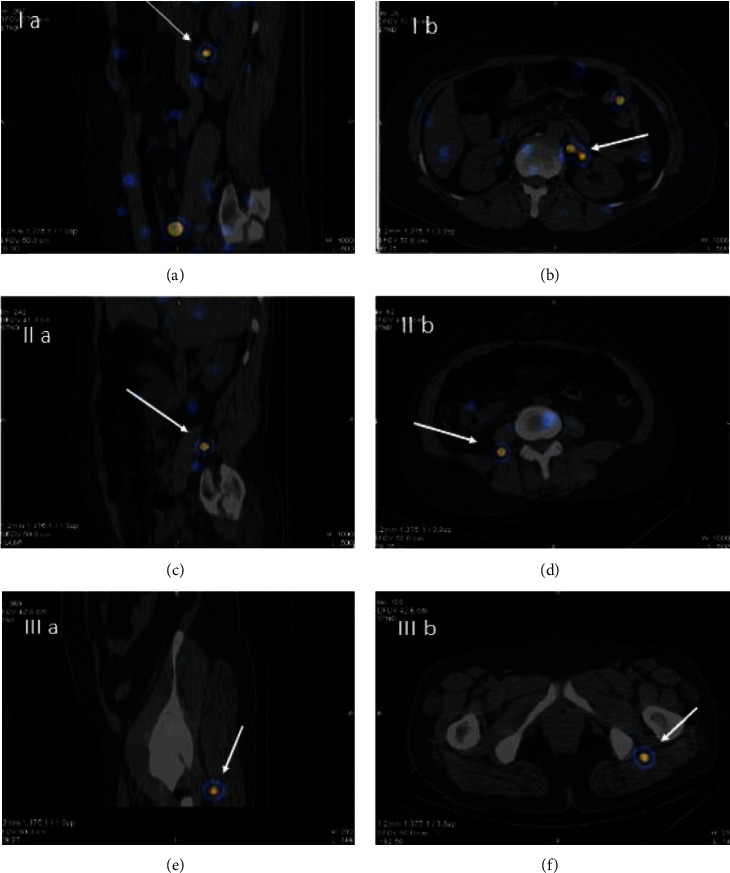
Aberrant ovarian sentinel lymph nodes (SLN) in the sagittal and transverse SPECT/CT lymphoscintigraphy. (a) and (b): intergluteal SLN; (c) and (d): peri-renal SLN; and (e) and (f): psoas region SLN.
